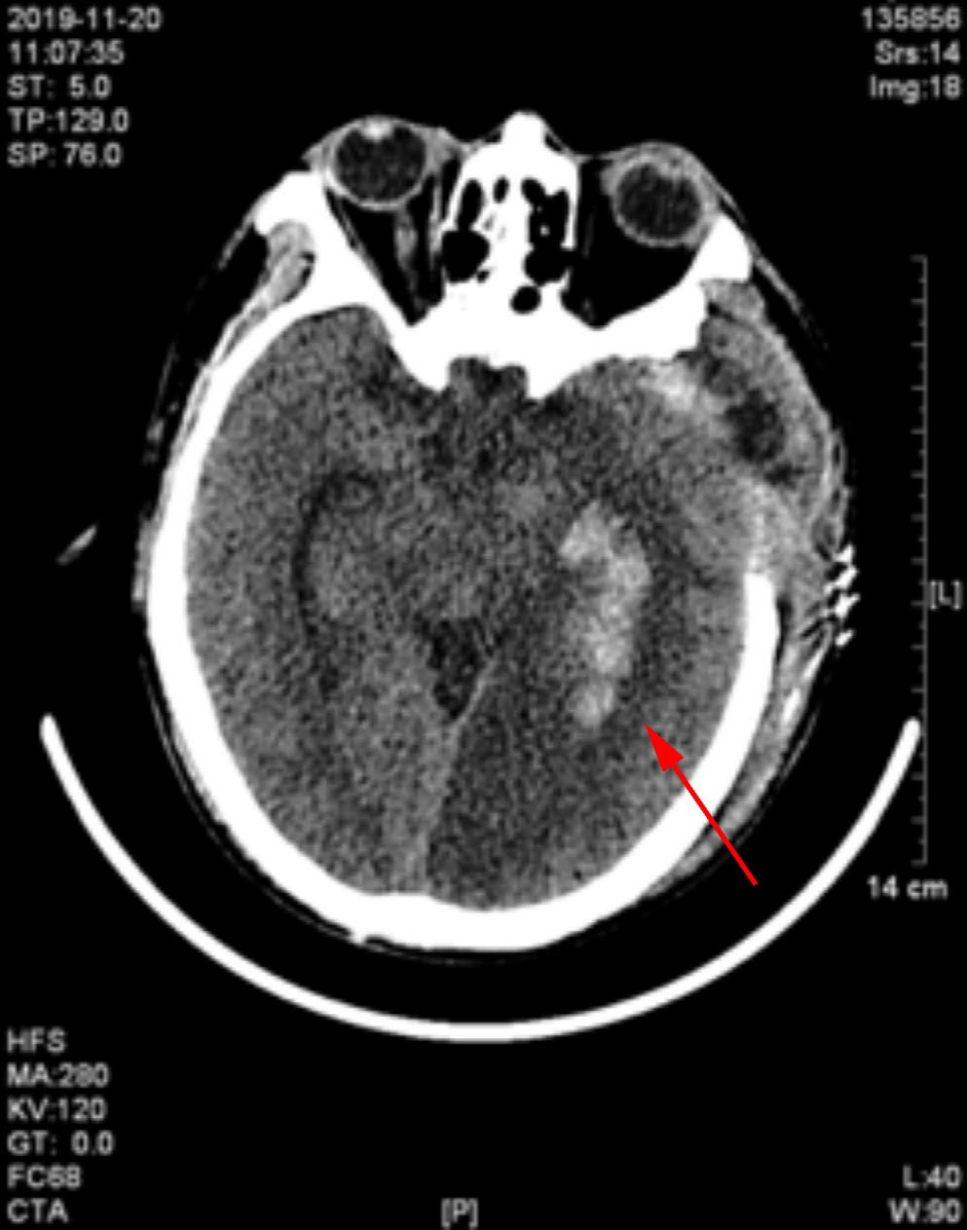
The Head CT revealed new intracranial hemorrhage on ICU day 10 (red arrow)
